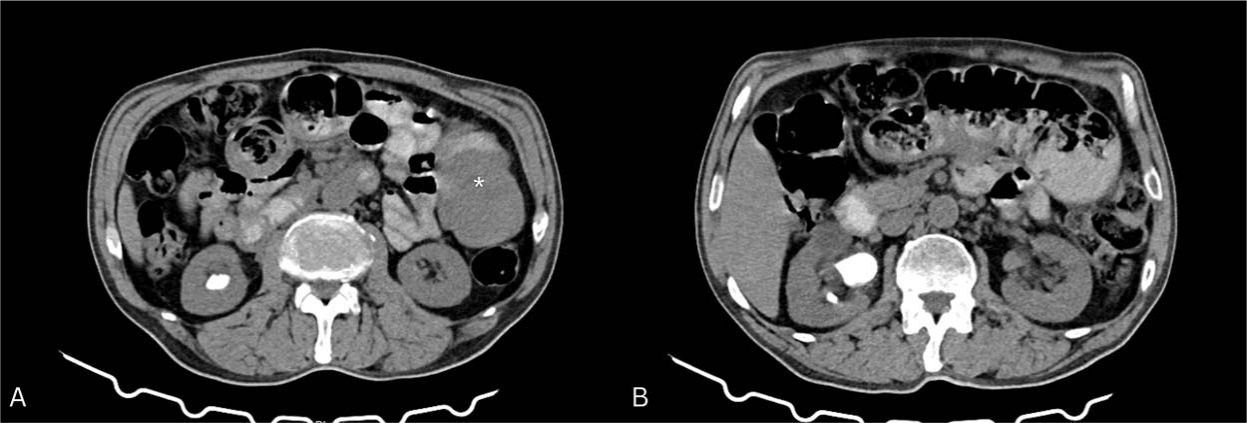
Contrast-enhanced CT of the abdomen. (A) Axial view showing a soft tissue mass-like lesion in the left abdomen (star). (B) Dilated bowel loops with mild to moderate thickening of the bowel wall.
